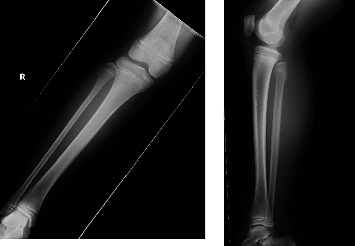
Radiograph of the right knee showing osteochondroma of the fibular head.
